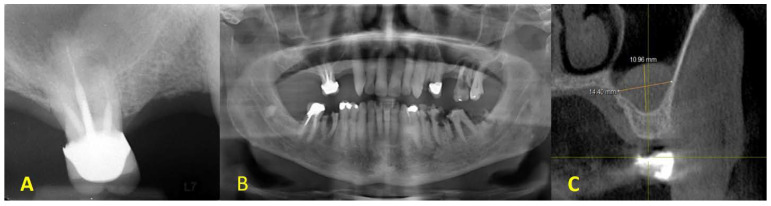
Preoperative radiographic evaluation: (A) homogenous solitary radiopaque mass observed on the right sinus floor in intraoral periapical with a periapical lesion on the MB root of the right maxillary molar; (B) a panoramic radiograph prior to initial therapy; (C) CBCT cross-sectional view of mucocele/antral pseudocyst with a patent osteomeatal complex.
